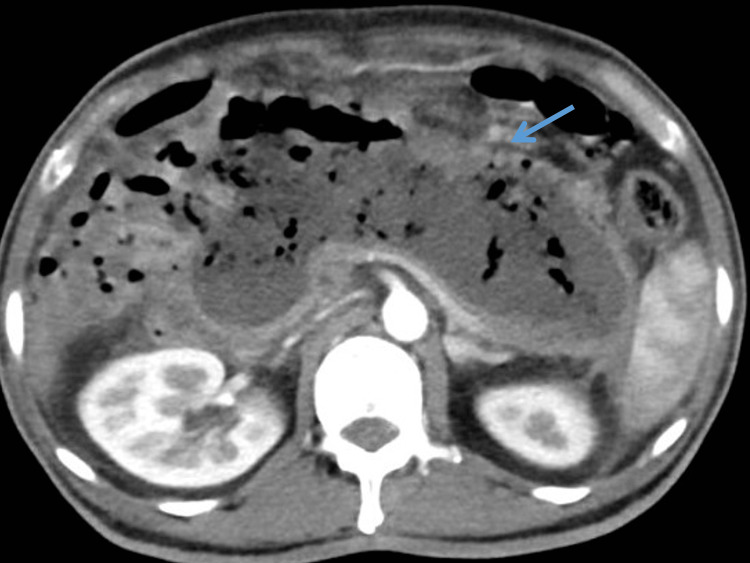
Non-contrast computed tomography (NCCT) of abdomen revealing 14 X 10 X 8 cm heterogenous collection in lesser sac with air pockets.
